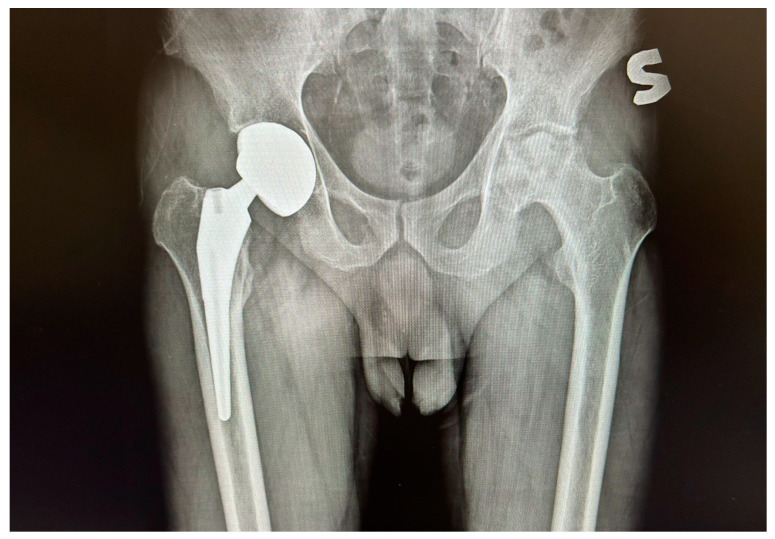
X-ray showing right implant after first THA.
